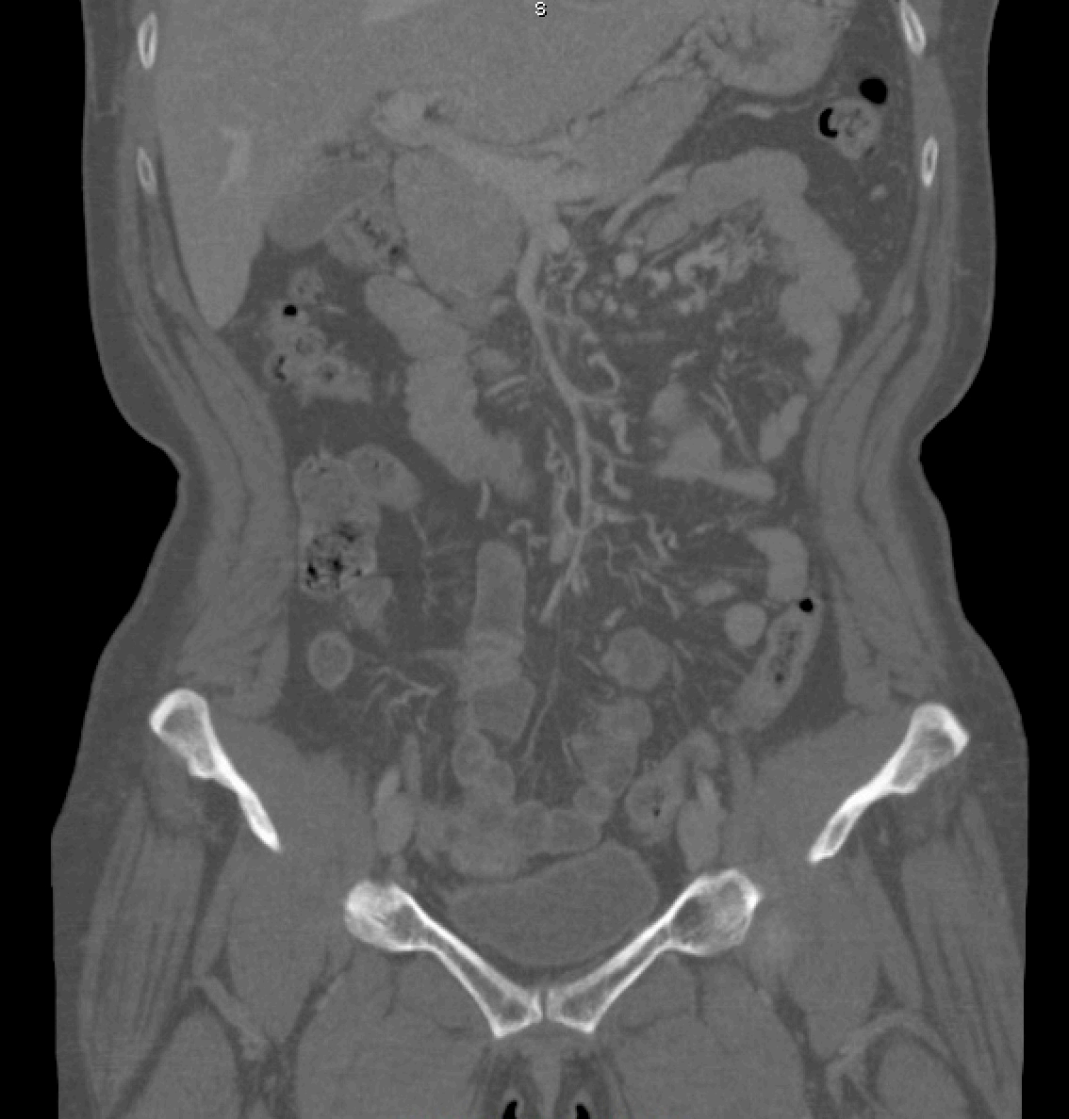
Computed tomography angiography of the abdomen and pelvis 6 months after treatment with anticoagulation, showing no filling failures– case 2.
